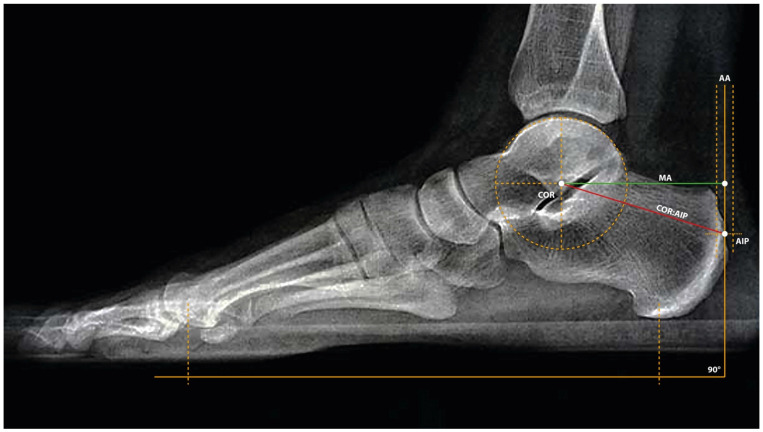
Lateral X-ray of the foot in a neutral position. The following were determined: COR—the center of rotation of the ankle joint; PP—the plantar plane as a line connecting the lower contour of the heel and the head of the first metatarsal bone; AA—the Achilles tendon axis as a line perpendicular to SP, passing through the center of the tendon; AIP—the tendon insertion point as the intersection of AA with the cortical outline of the calcaneal tubercle; MA (green line)—the lever arm as the distance from COR to the intersection of AA, perpendicular to SP passing through COR.
